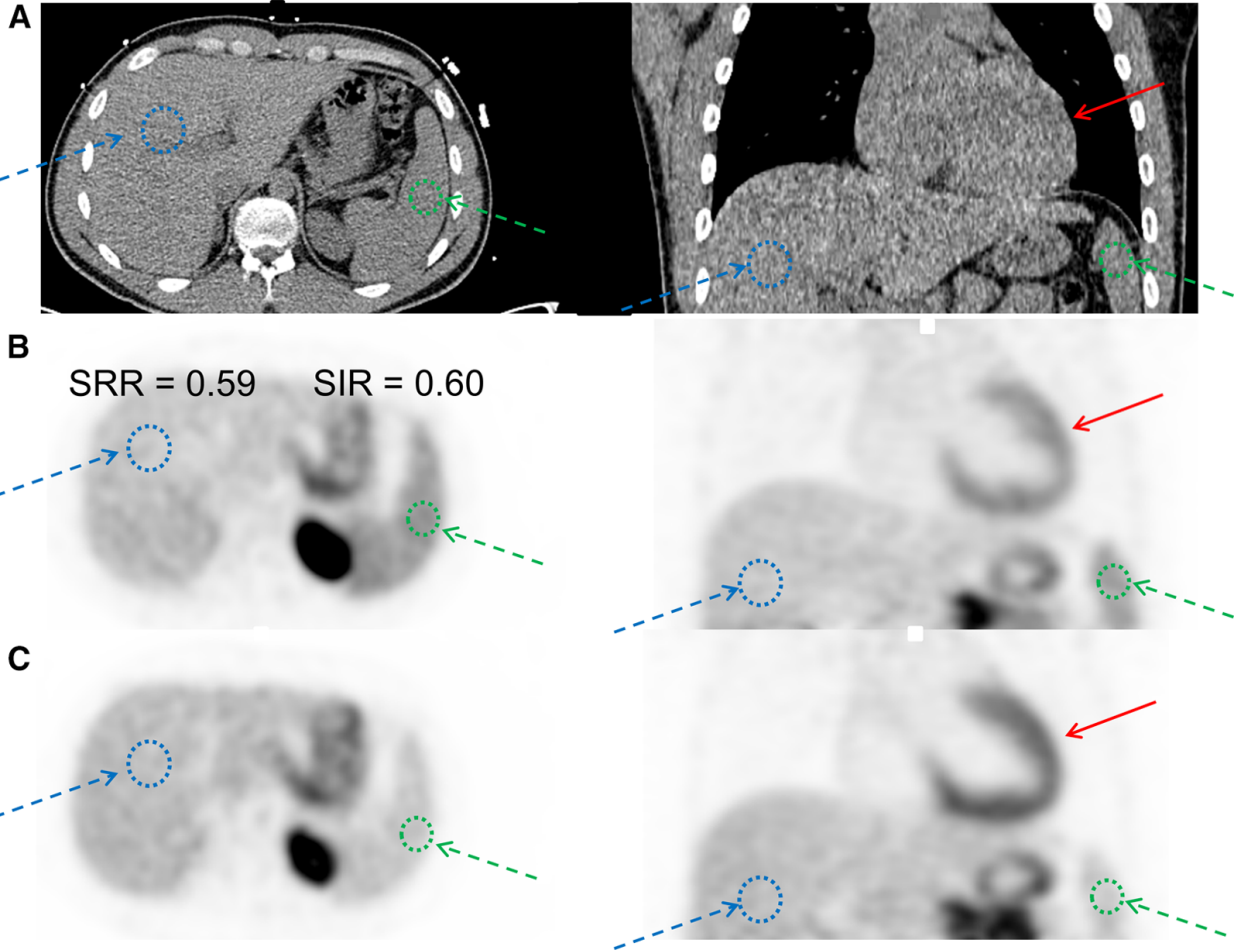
Identification of SRR and SIR. Two volumes of interest were in the spleen (sphere, *r* = 20 mm − green arrow) and liver (sphere, *r* = 50 mm − blue arrow). Anatomical identification of the spleen and heart (red arrow) was obtained using the low-dose CT images acquired for attenuation correction purposes (a). In b and c we show the rest and stress uptake parameters for a scan with concordant assessment of adequate hyperemic response as obtained using both SRR and SIR. *SRR* splenic response ratio, *SIR* splenic stress-to-rest intensity ratio
